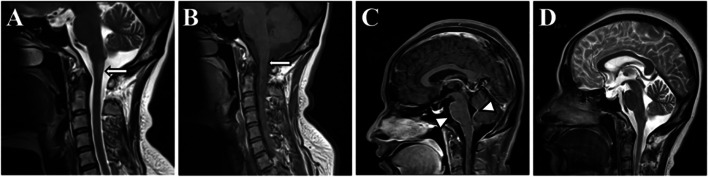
Sagittal MRI shows abnormal signal intensities in the dorsal medulla oblongata including area postrema (arrows), with hyperintense on T2-weighted image and hypointense on T1WI (A, B). Sagittal T1-weighted postgadolinium imaging demonstrates linear enhancement along the surface of the brainstem and fourth ventricle (C, arrowheads). Sagittal T2-weighted imaging reveals resolution of T2-hyperintense lesion (D)
